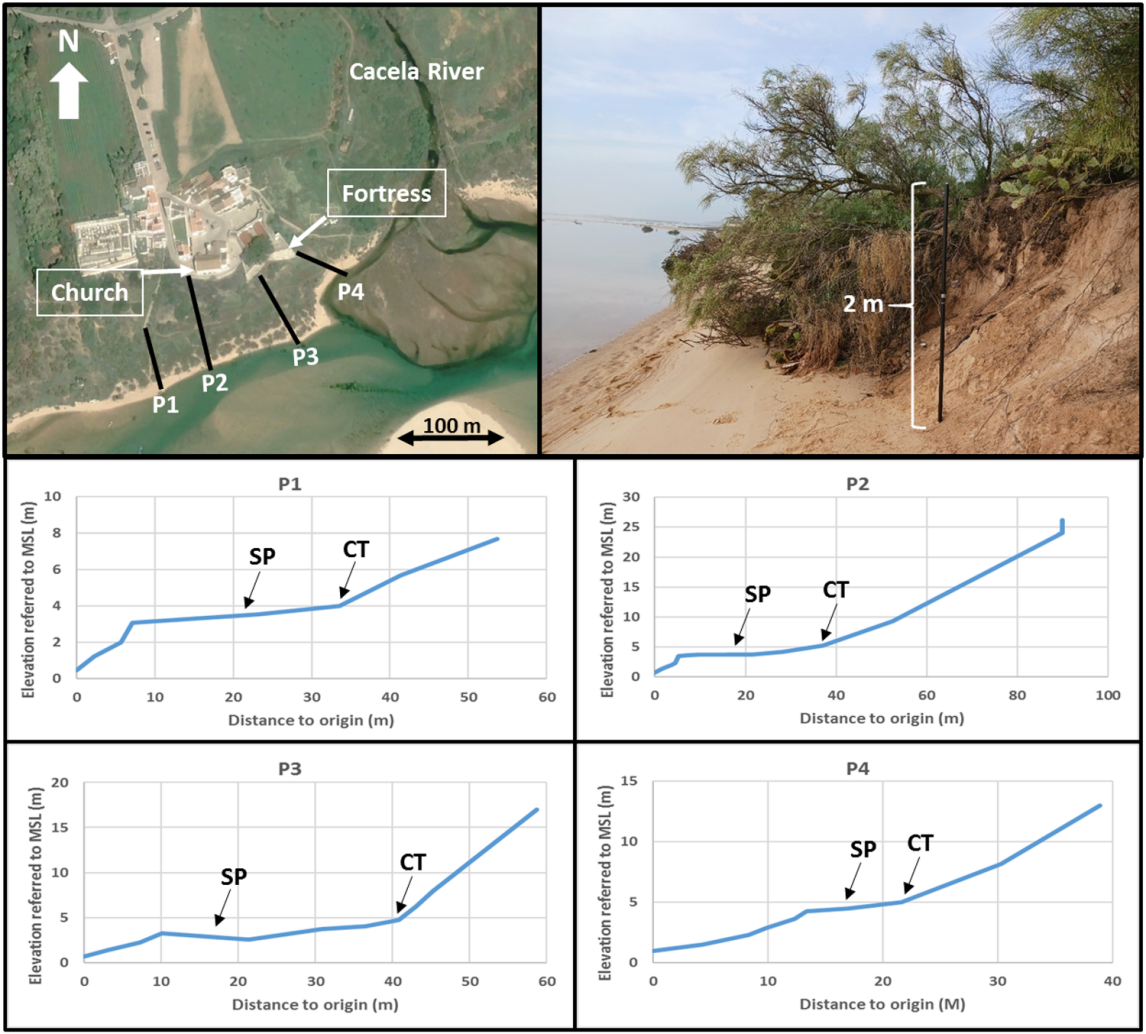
Location of the topographic profiles (P1 to P4, top left panel), evidence of strong retreat by scarp formation and consequent vegetation fall (top right panel), and topographic profiles from the lagoon (origin) to a mid or upper cliff position (lower panels). SP—Sandy platform, CT—Cliff toe. Aerial image source: Google Earth, Airbus, April 2020.
